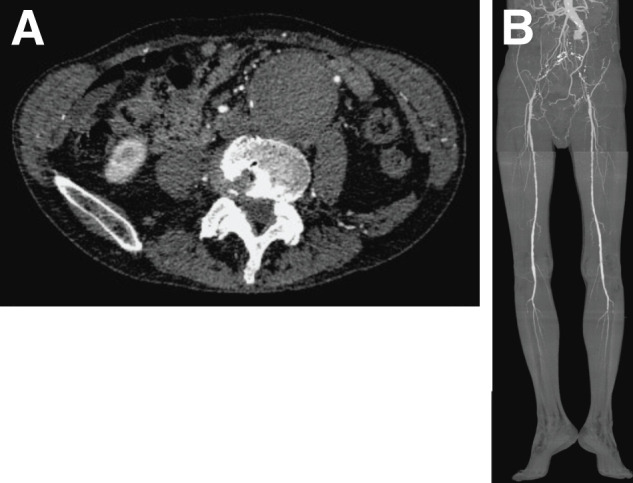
Preoperative CTA images. (A) A 53-mm AAA with complete thrombosis of the aneurysm lumen. (B) 3D maximum intensity projection image. AAA, abdominal aortic aneurysm
